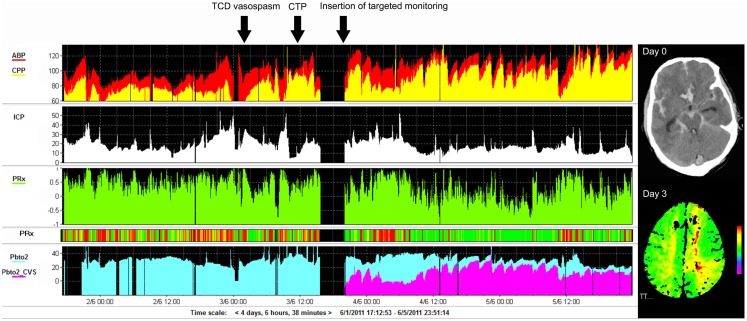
Example of monitoring and imaging data from a patient with SAH (WFNS 5) with an AComA aneurysm, which was clipped on day 2 post-ictus. Invasive monitoring probes including ICP and PbtO_2_, as well as an EVD, were inserted on the right side on admission. During the course of treatment, the patient developed cerebral vasospasm, which was initially detected using TCD monitoring on day 3 post-ictus (black arrow). Subsequent CTP on day 4 demonstrated a perfusion deficit in the left ACA territory (second black arrow signifies the time CTP performed), i.e., contralateral to the PbtO_2_ probe (importantly, the PbtO_2_ values were within normal range at all times, i.e., >25 mmHg and further responded to hypertension, rising to >30–35 mmHg). A further PbtO_2_ probe, targeted to the area of perfusion deficit seen on CTP, was inserted (third black arrow indicates time of insertion), which demonstrated lower/ischemic (<15 mmHg) values of PbtO_2_ (purple color on graph). ACA, anterior cerebral artery; AComA, anterior communicating artery; CTP, CT perfusion; EVD, external ventricular drain; PbtO_2_, brain tissue oxygen; SAH, subarachnoid hemorrhage; TCD, transcranial Doppler; WFNS, world federation of neurosurgical societies scale.
